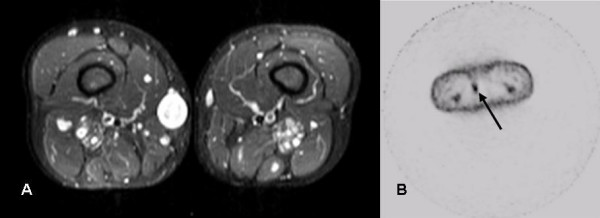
A) Transaxial MRI scan (STIR, 5 mm slice thickness, left) and B) Corresponding FDG-PET scan (5 mm slice thickness, right). Increased FDG uptake is visible in a subcutaneous lesion located medially in the right upper leg (arrow).
